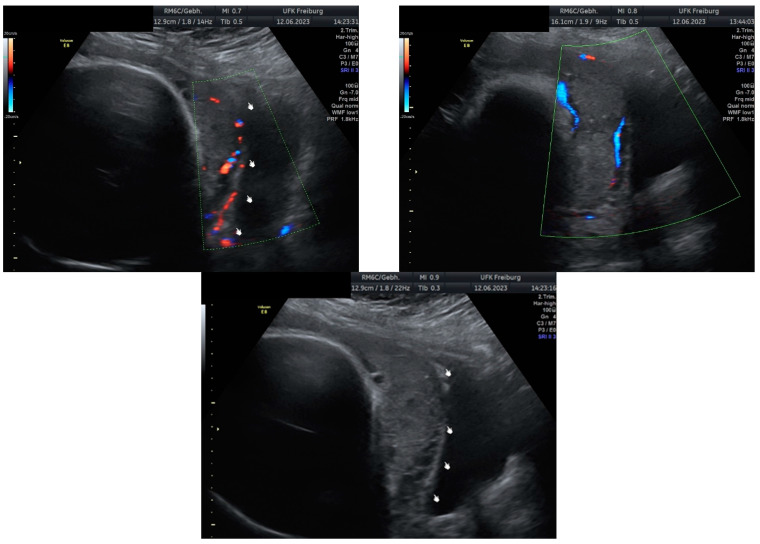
According to the standardized description of the European Working Group on Abnormally Invasive Placenta for ultrasound anomalies, the following anomalies may be seen: Loss of the “clear zone”—partially more in the middle (N/3):
